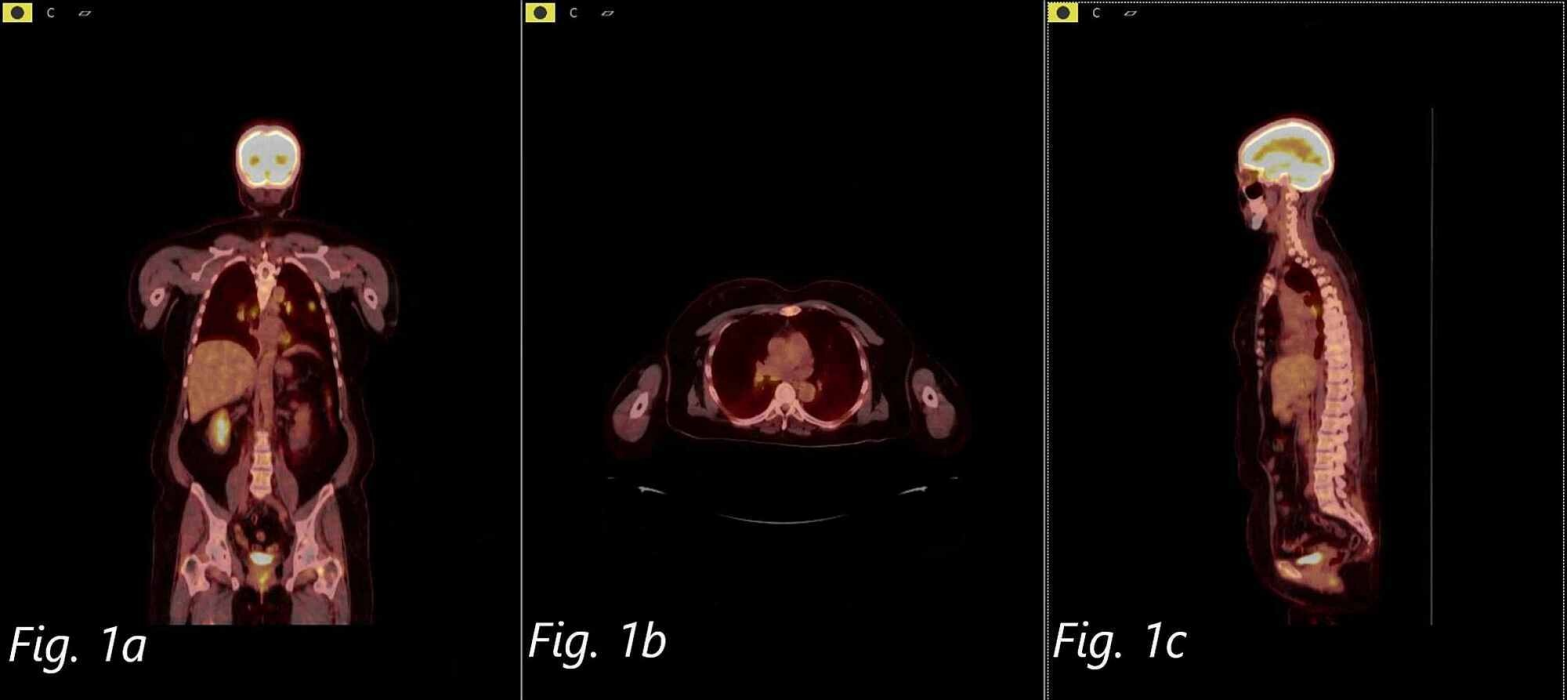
Coronal (a), axial (b), and sagittal (c) views of FDG PET/CT scan whole body showing multiple areas of hypermetabolic activity indicative of metastasis. FDG: Fluorodeoxyglucose; PET: Positron emission tomography.
